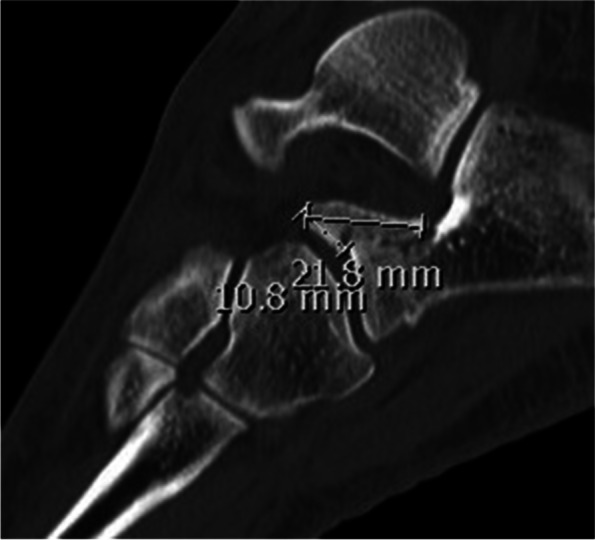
Measuring the fragment size in the sagittal CT with an estimated digital measurement tool (Impax, Agfa, Germany)
